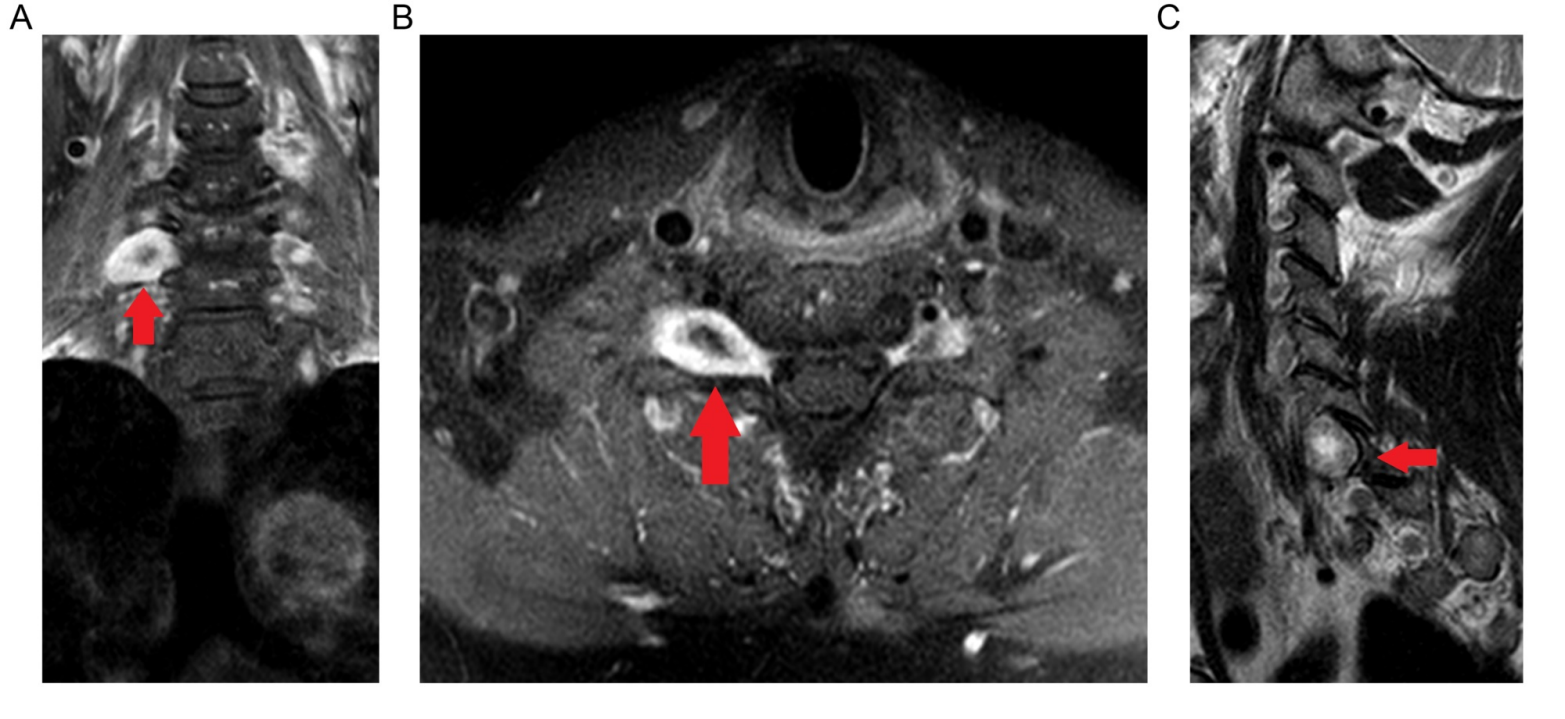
Gadolinium-enhanced T1-weighted coronal (A), axial (B), and T2-weighted sagittal (C) MRI signal highlighting a centrally cystic/necrotic nerve sheath tumor at the level of C7 (arrows).
